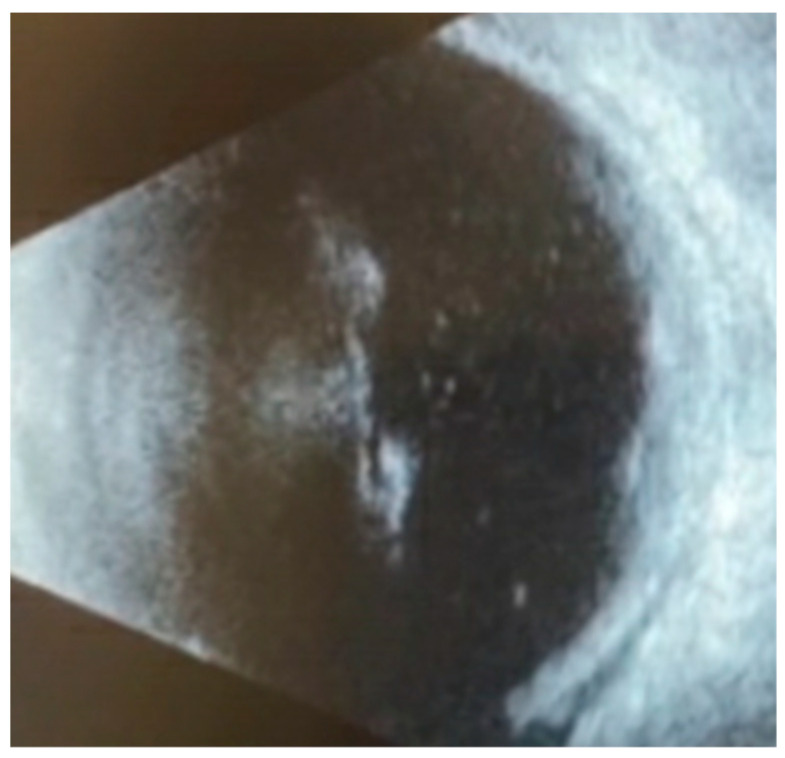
B-scan ultrasonography of macular lesions in the left eye on initial examination demonstrated vitreous opacities consistent with vitritis, elevated subretinal lesions, and a thickened choroid.
